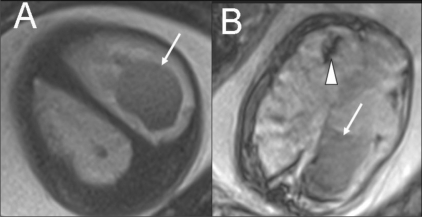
Single live gestation at 33 weeks. T2W (A) and gradient-echo T2W (T2*) MRI images show intraventricular (arrows) and periventricular (arrowhead) hemorrhage. Fetal blood sampling was negative for TORCH IgMs. A platelet count of 50,000 / mm3 was noted. Pregnancy ended with a stillbirth at 35 weeks.
